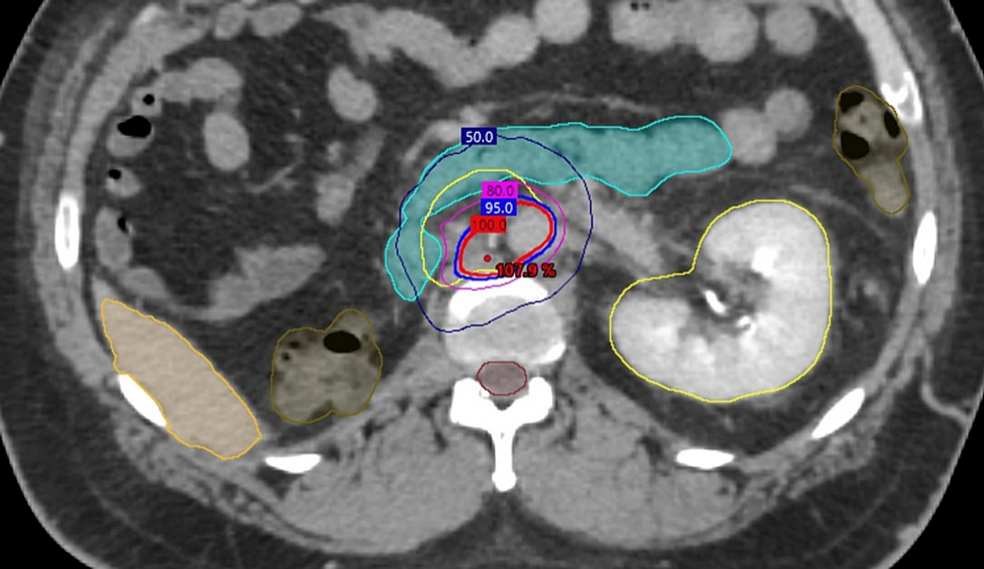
Radiotherapy treatment plans for oligometastatic papillary renal cell carcinoma (pRCC) of the retroperitoneal lymph nodes Radiotherapy treatment planning computed tomography (CT) axial images are displayed for the management of pRCC involving the retroperitoneal nodes. The prescription dose is represented by the 100% isodose line (red) to a total of 60 Gy in 15 fractions. Regional hotspots are noted as point doses (red) with additional isodose lines of 95% (blue), 80% (pink), and 50% (navy). Nearby organs at risk (OARs) are contoured for (A): trachea (orange), esophagus (cyan), left brachial plexus (lime), spinal cord (salmon), and (B): liver (orange), duodenum (cyan), large bowel (brown), left kidney (yellow), spinal cord (burgundy).
